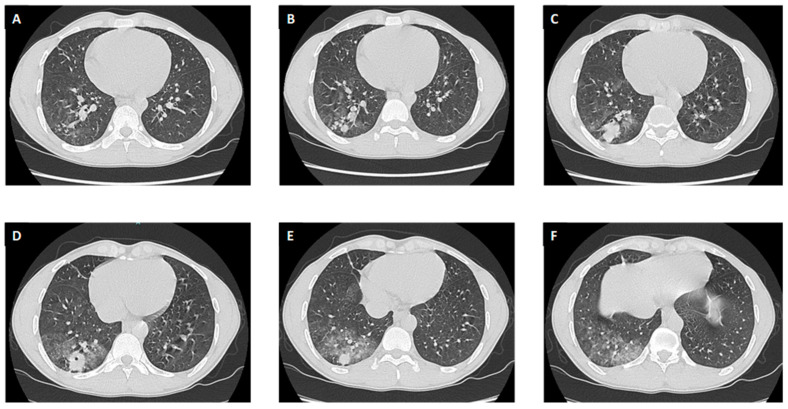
Chest CT scan at presentation (Panel (A–F)).
